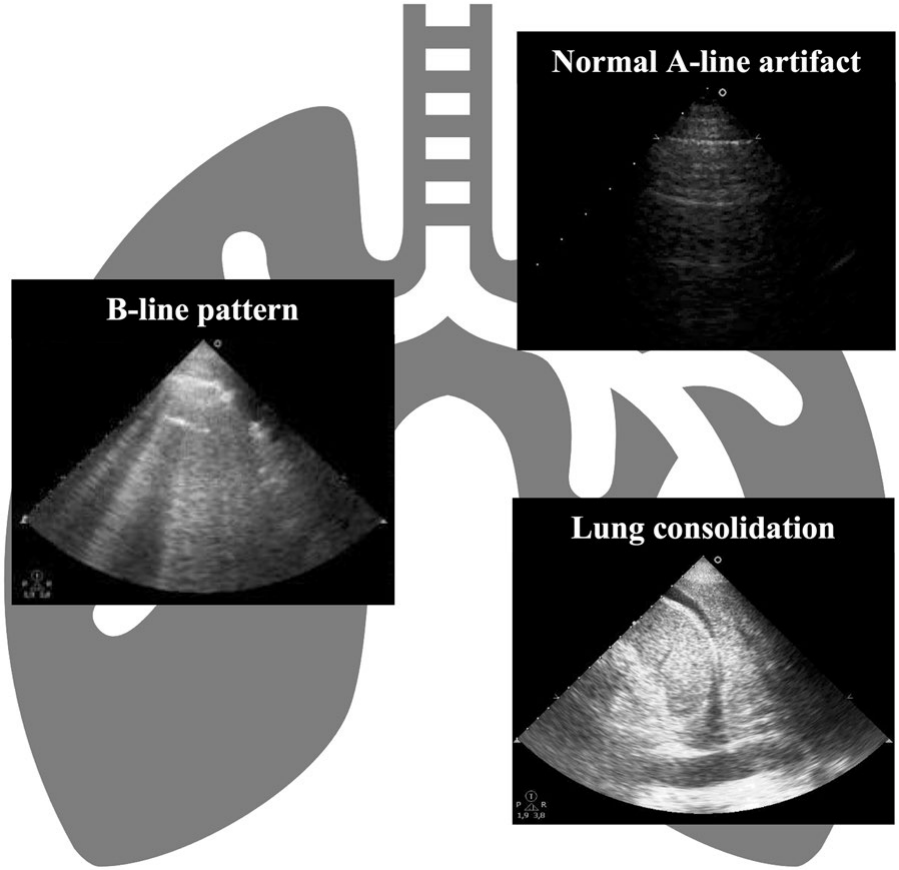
Different echographic patterns during lung ultrasound evaluation
